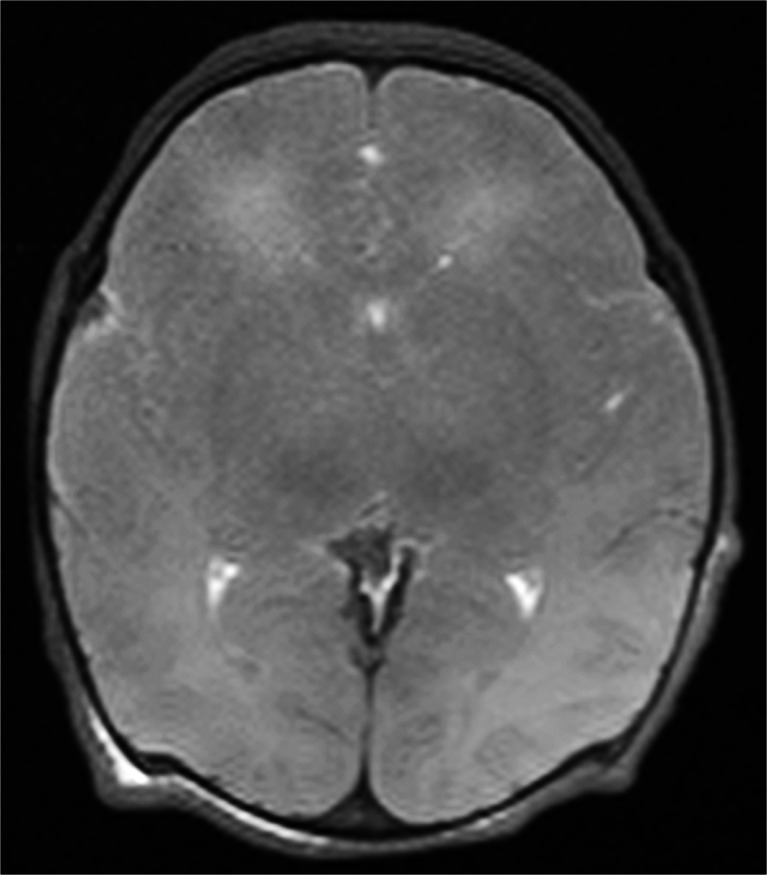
Brain ischaemia. Axial T2-weighted postmortem magnetic resonance imaging of the brain in a term baby found abandoned. There is gross cerebral oedema with loss of sulcal definition, and almost complete loss of the grey-white matter interface. It is not currently possible to differentiate antemortem from postmortem hypoxic-ischaemia brain injury on conventional postmortem magnetic resonance imaging sequences
